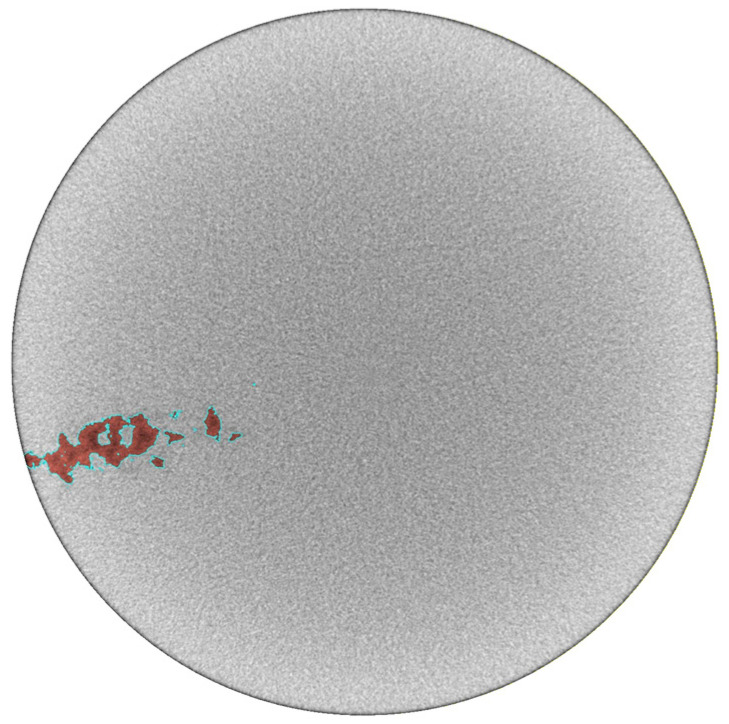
2D slice of the maximum cross-sectional area $a_{\mathrm{CT}\max}$ of the outlier defect.
